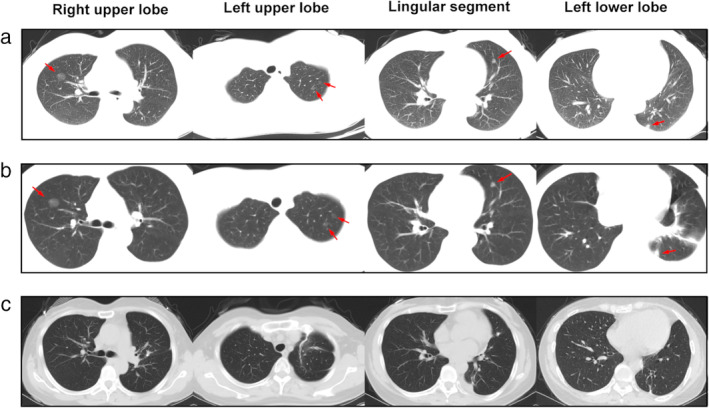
(a) CT image after trauma showed a pure ground‐glass nodule (pGGN) of approximately 14 mm in the right upper lobe, two pGGNs of approximately 6 mm in the apical segment of the left upper lobe, a mixed GGN (mGGN) of approximately 8 mm in the left lingular segment, and a mGGN of approximately 8 mm in the left lower lobe. (b) There were no significant changes in the nodules after one week of anti‐inflammatory treatment. (c) The pGGN in right upper lobe had disappeared. Shadows of staples and postoperative changes can be seen in the left upper and lower lobes.
